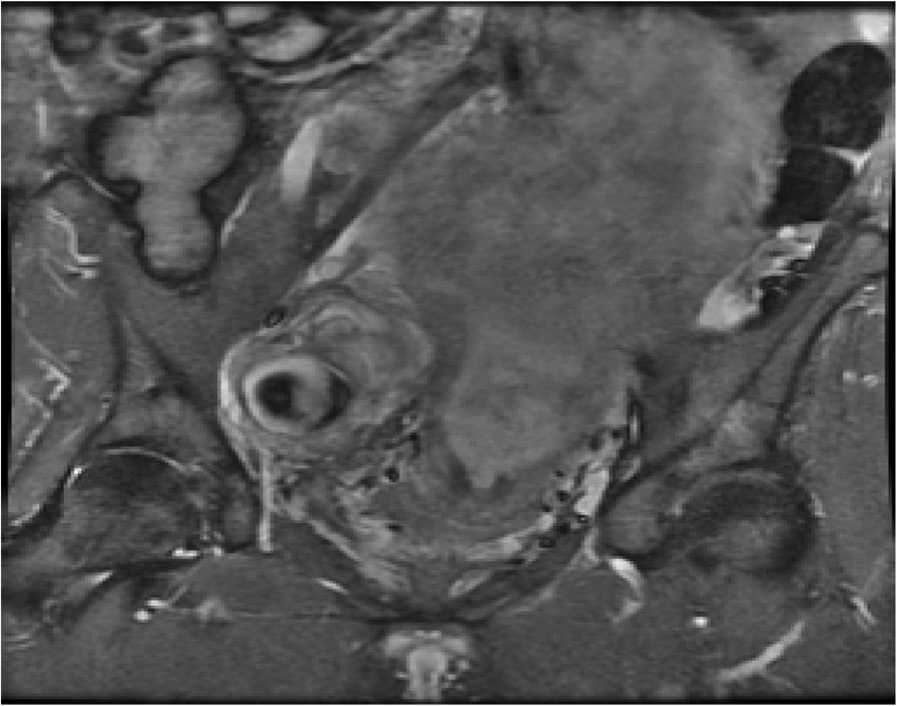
MRI: right uterine artery pseudoaneurysm
